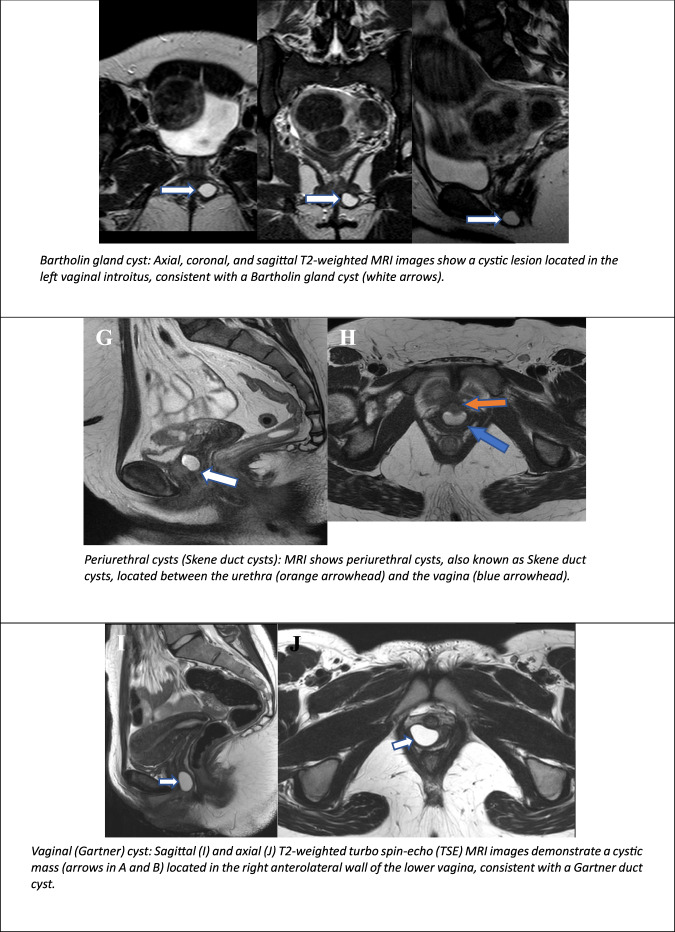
Non-ovarian cystic female pelvic lesions. Pelvic cystic schwannoma: Axial T2-weighted TSE MRI image (A) shows a heterogeneous mass adjacent to the right sciatic foramen (arrow), with predominantly cystic components. Corresponding post-contrast T1-weighted fat-suppressed (T1 FS TSE) MRI image (B) reveals enhancing solid components within the lesion (arrow), characteristic of a cystic schwannoma. Cystic Gastrointestinal Stromal Tumours (GISTs): a woman presented with pelvic pain. Axial T2WI (C) demonstrates a predominantly solid mass (asterisk) attached to the LT postmenopausal ovary (arrow). Also shown is the close relation of the mass with the adjacent small bowel loops (arrow in D). Gastrointestinal stromal tumour Tarlov Cyst: Sagittal T2-weighted MRI (E) and axial T2-weighted MRI (F) images demonstrating a Tarlov cyst (blue arrow), a cerebrospinal fluid-filled perineural cyst commonly found in the sacral region. Bartholin gland cyst: axial, coronal, and sagittal T2-weighted MRI images show a cystic lesion located in the left vaginal introitus, consistent with a Bartholin gland cyst (white arrows). Periurethral cysts (Skene duct cysts): MRI shows periurethral cysts, also known as Skene duct cysts (G), located between the urethra (orange arrowhead) and the vagina (blue arrowhead) (H). Vaginal (Gartner) cyst: sagittal (I) and axial (J) T2-weighted turbo spin-echo (TSE) MRI images demonstrate a cystic mass (arrows in I, J) located in the right anterolateral wall of the lower vagina, consistent with a Gartner duct cyst
